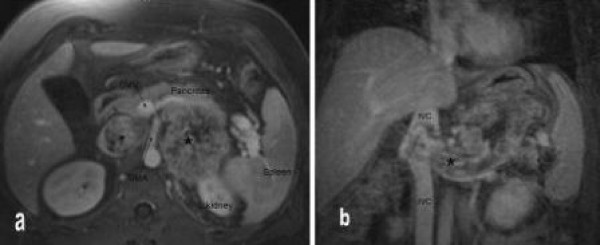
a, Magnetic resonance imaging shows the tumor (large asterisk) lying to the left of the superior mesenteric artery (SMA), involving the distal pancreas anteriorly, the superior pole of the left kidney posteriorly, and extending into the inferior vena cava (IVC) (small asterisk). b, The tumor (large asterisk) involves and encases the left renal vein and extends into the IVC. SMA indicates superior mesenteric artery.
